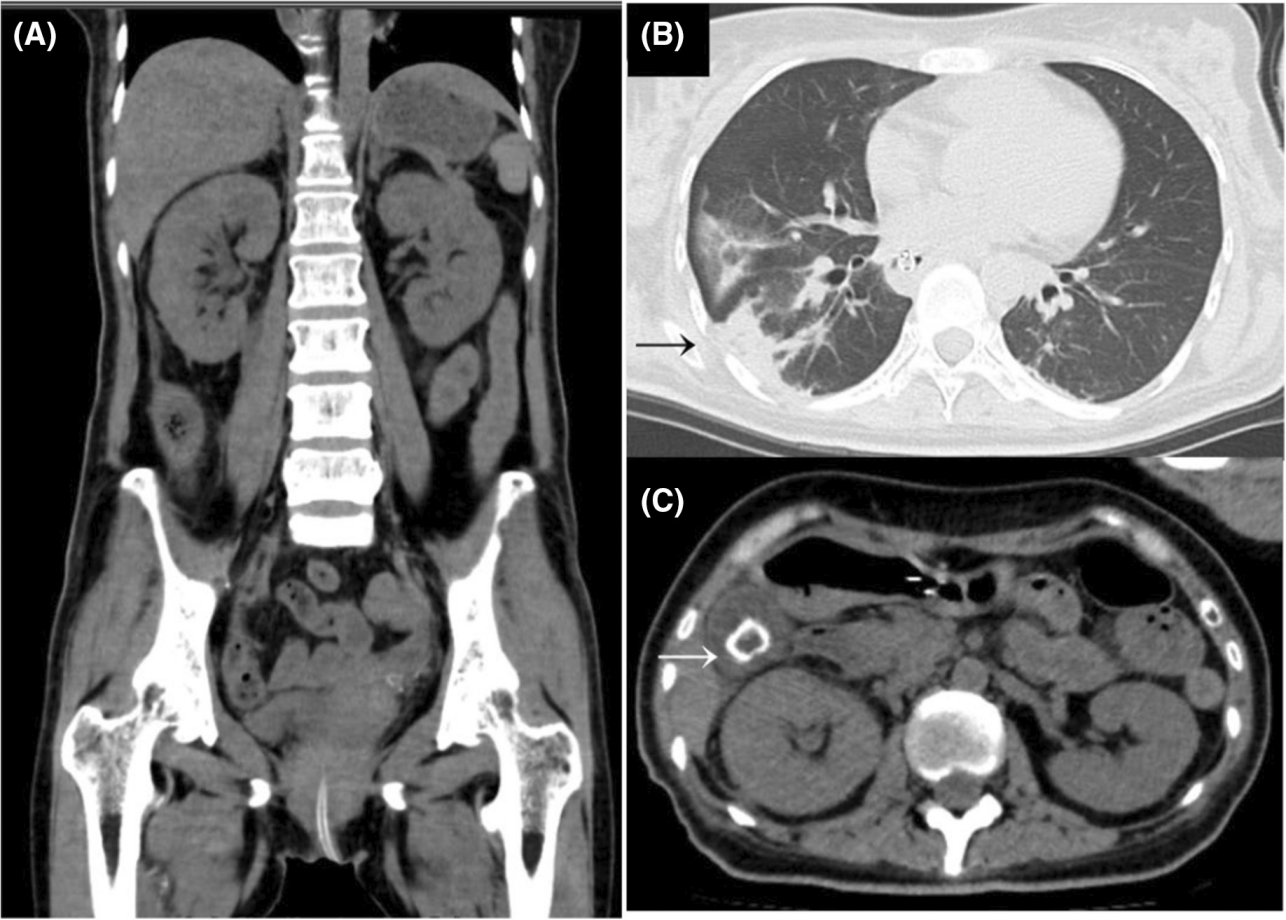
Computed tomography radiographs of a patient after arriving at the hospital. There are no obvious abnormalities in the urinary tract (A–C) other than moderate pneumonia (black arrow) (B) and gallstones (white arrow) (C).
